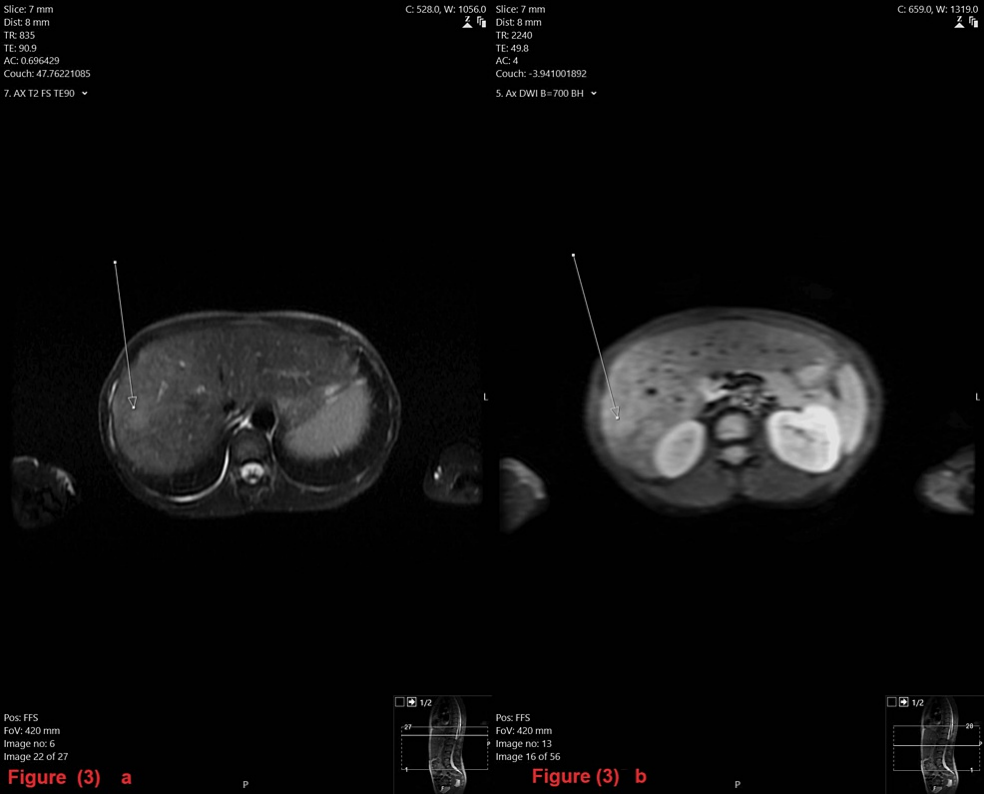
3a: MRI of the patient's abdomen (axial view) T2 weighted image (T2W1); Figure 3b: MRI of the abdomen (axial view) 3a: An MRI of the abdomen (axial view) T2WI revealed multiple lesions in the liver with hyperintensity, which appeared confluent in segments 7/8; 3b: An MRI of the abdomen (axial view) revealed multiple areas of restricted diffusion corresponding to the same lesions in the liver.
